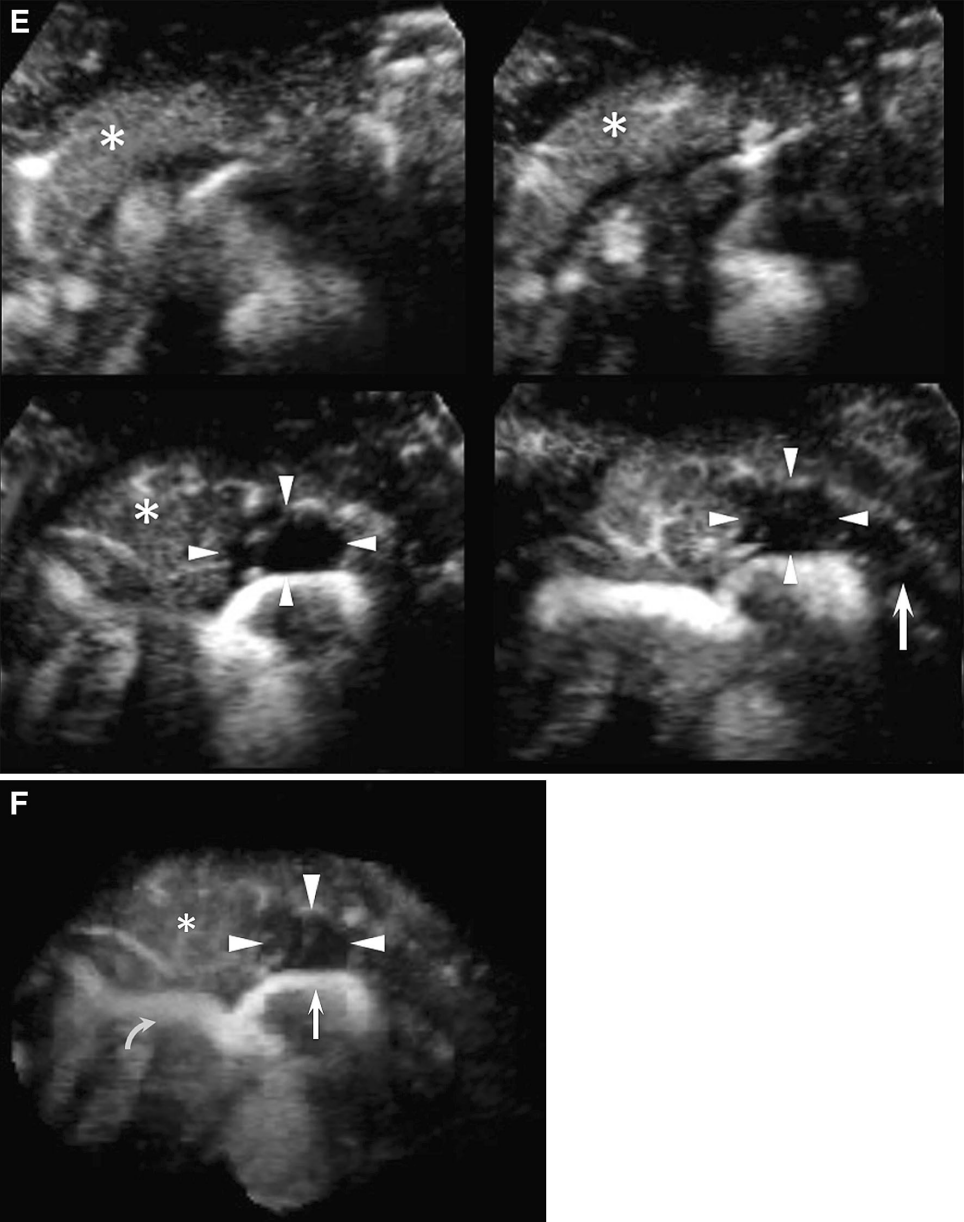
CE 3D US images acquired in the transverse plane of a 43-year-old man with PDAC (maximum diameter, 38 mm) in the pancreatic body. A–D Hypovascular lesions (*arrowheads*) are clearly seen in three orthogonal planes in the arterial phase. *Small green squares* show the center of a lesion. (A An image for navigation of the following planes; B plane A, from the front to the back; C plane B, from the left to the right; and D plane C, from the top to the bottom). E TUIs of CE 3D US (plane A) in the arterial phase show a hypovascular lesion (*arrowheads*) compared with the parenchyma in the pancreatic head (*asterisks*) with peritumoral and fine vessels. The dilated main pancreatic duct appears as a non-enhanced area in the caudal part of the lesion (*arrow*). F Sonographic angiogram in the arterial phase, rendered in the average intensity mode, shows the hypovascular lesion in the pancreatic body (*arrowheads*) compared with the parenchyma (*asterisk*). The splenic artery with encasement by tumor invasion (*arrow*) and common hepatic artery (*curved arrow*) is clearly seen posterior to the pancreas.
